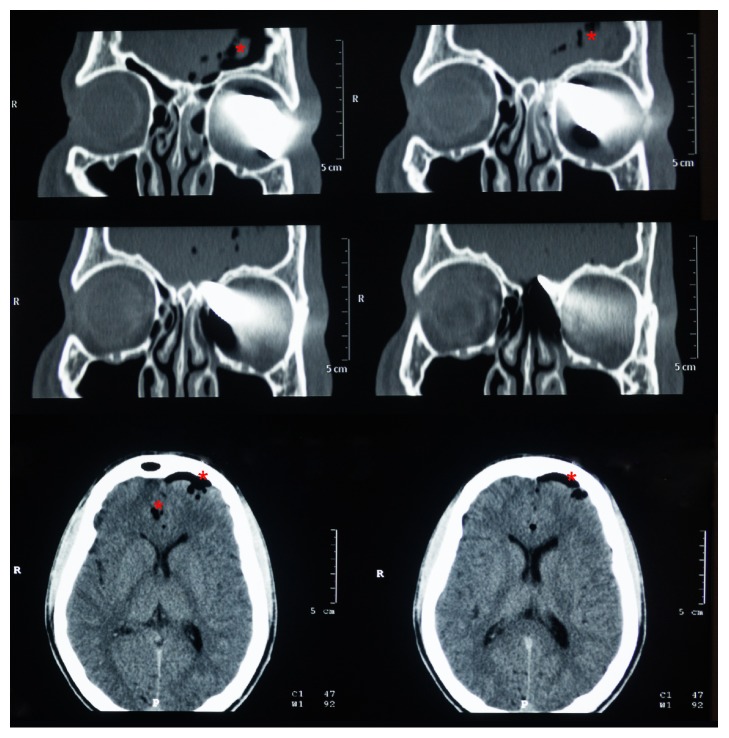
CT scans showing intraorbital foreign body with triangular shape of 4.6 cm x 2 cm in the left orbit, passing through the orbit and the ocular globe, fracturing the superomedial wall of the orbit, with a probable associated fracture of the ethmoidal cells, and reaching the anterior cranial fossa, causing pneumocephalus (marked by asterisks).
